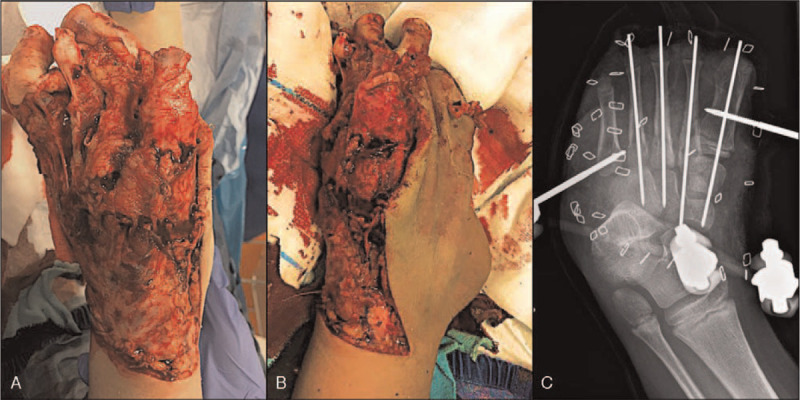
Pediatric degloving foot injury. (A) and (B) Preoperative dorsal and medial view revealing devascularized toes and extensive dorsal and plantar bone and soft tissue defects. (C) X-ray after toe amputation and temporary bone stabilization with K-wires and external fixation.
